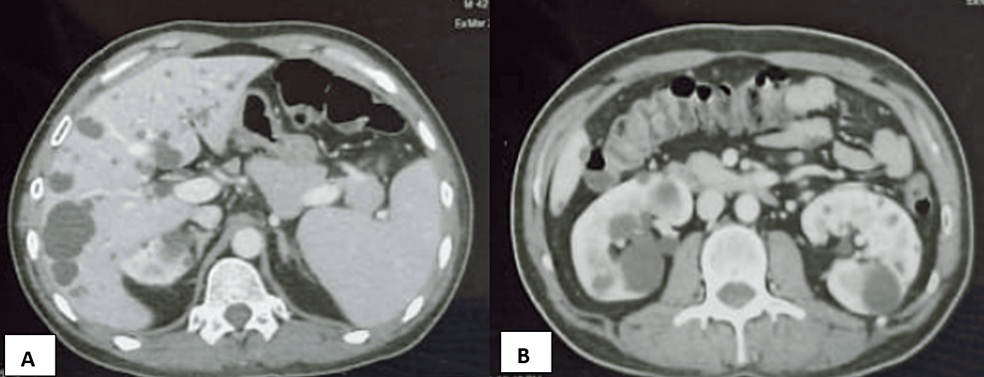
CECT images showing polycystic (A) liver and (B) kidney disease. CECT: Contrast-enhanced computed tomography
